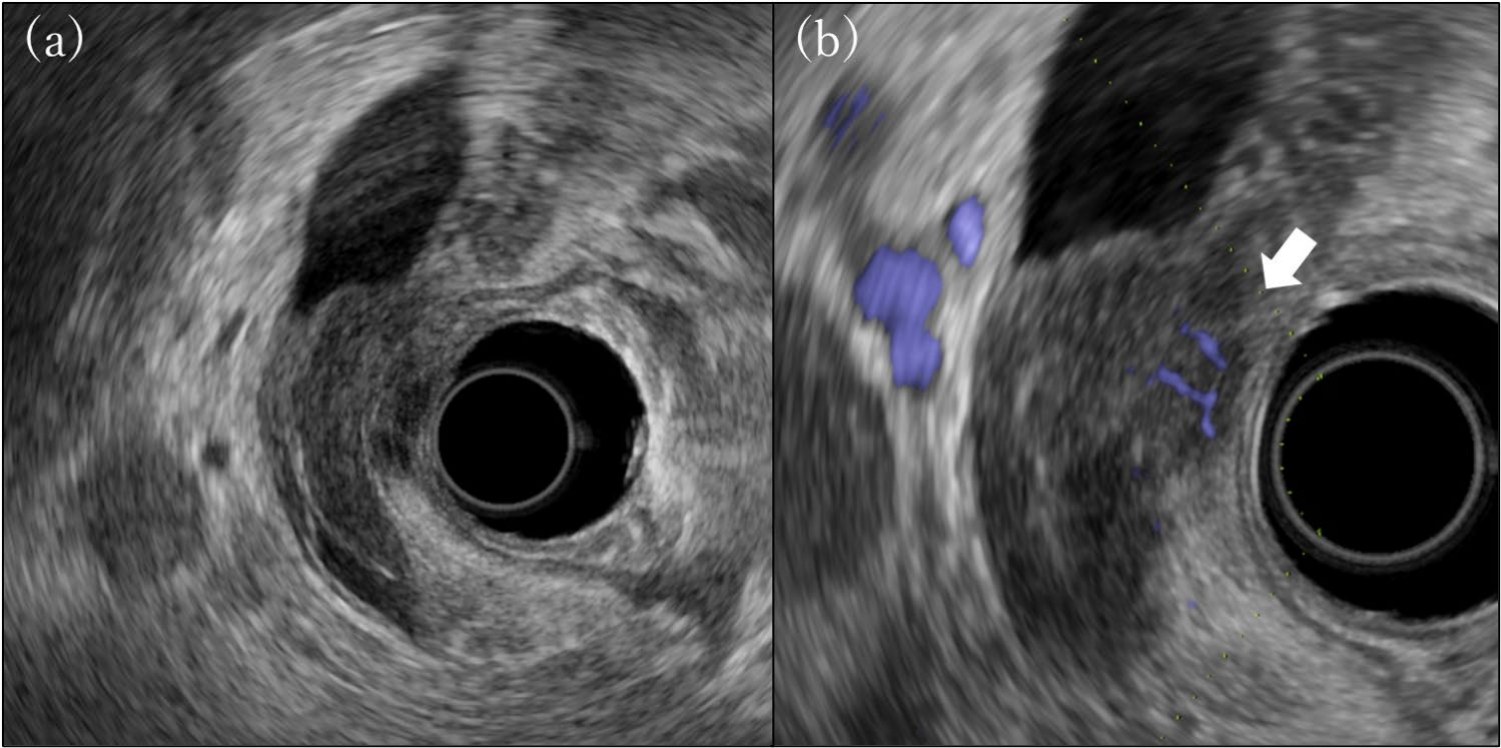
Endoscopic ultrasonography reveals wall thickening with a smooth mucosal side, but the outer high-echoic layer is partially disrupted (a). An abundant blood flow signal is seen in the outer irregular region (b) (arrow)
